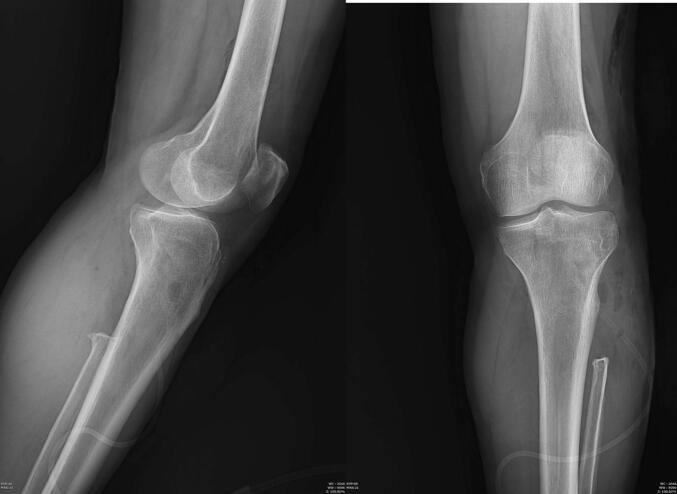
Postoperative plain radiograph following surgical resection of the proximal fibular lesion, confirming removal of the recurrent tumor.
